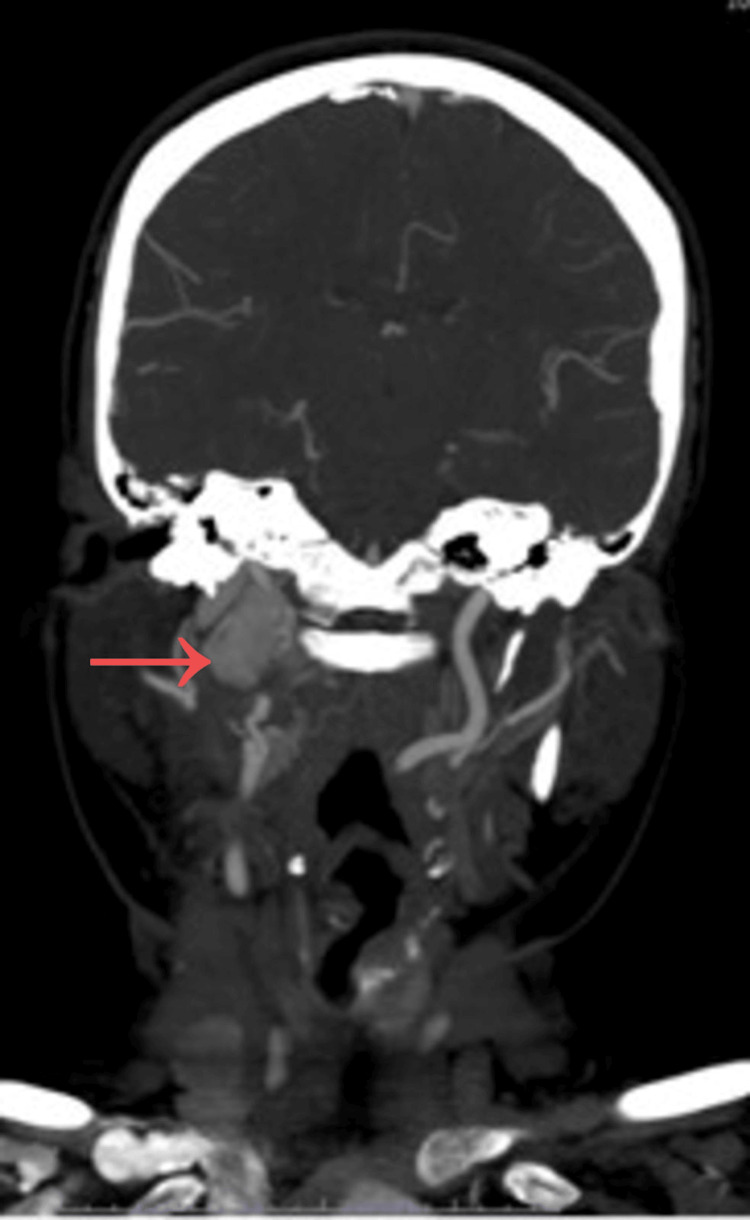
Coronal contrast-enhanced CT scan showing a well-defined mass in the right carotid space (arrow), extending along the vascular axis and causing lateral displacement of surrounding anatomical structures.
